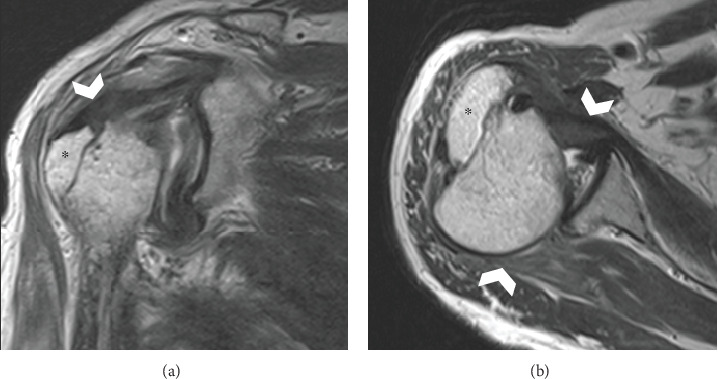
Preoperative MRI. (a) A coronal view showing an intact rotator cuff supraspinatus tendon. (b) The axial view showing an intact rotator cuff and subscapularis and infraspinatus tendons and no dislocation of the long head of the bicep tendon. Asterisk: greater tuberosity; white arrowhead: intact rotator cuff. MRI: magnetic resonance imaging.
